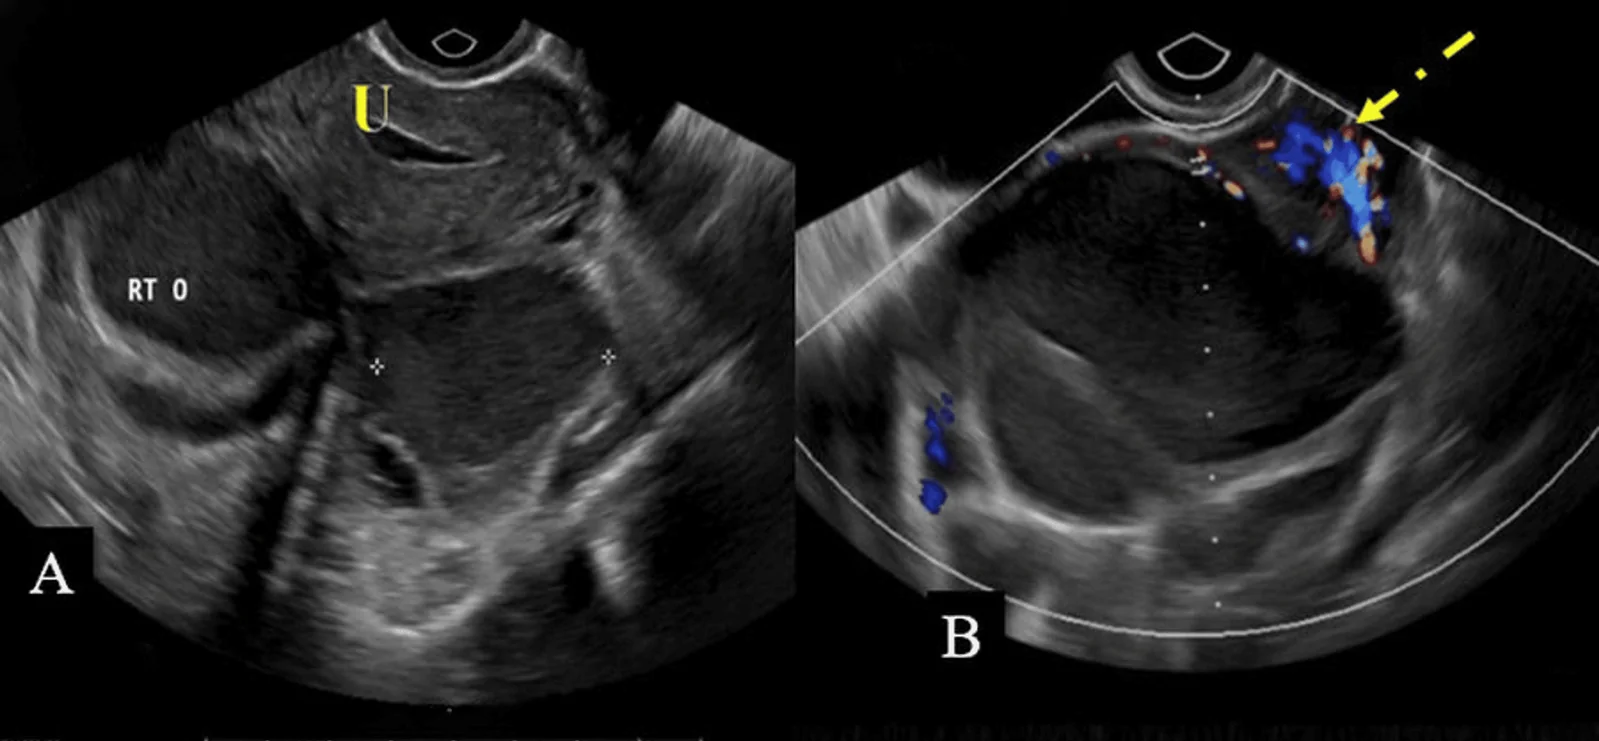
TVUS demonstrating bilateral ovarian abnormalities "A" shows both ovaries on a transverse scan, "Rt O" shows the right ovary, "U" shows the uterus, and "B" shows color Doppler imaging with vascular flow in the capsule (hatched yellow line) and no vascular flow within the ovary itself. The left ovary measures 3.74 cm. Low-level echoes within the ovarian tissue are consistent with hemorrhagic cysts or endometriomas. TVUS: transvaginal ultrasound
